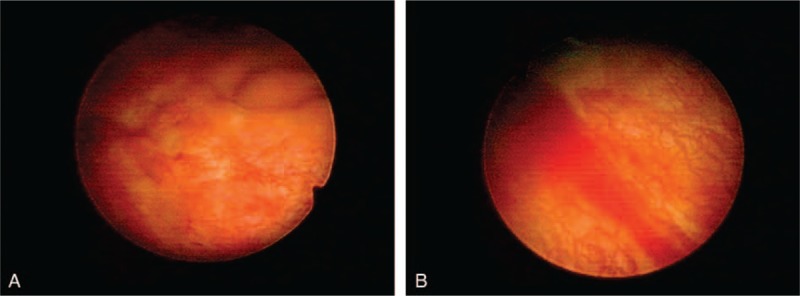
A, Irregular bladder mucosa surface with hump-like changes over the anterior aspect of the bladder wall without tumor lesions (location at which CT revealed one nodule). B, Easy bleeding after hydrodistension with glomerulation and ulcerative change.
